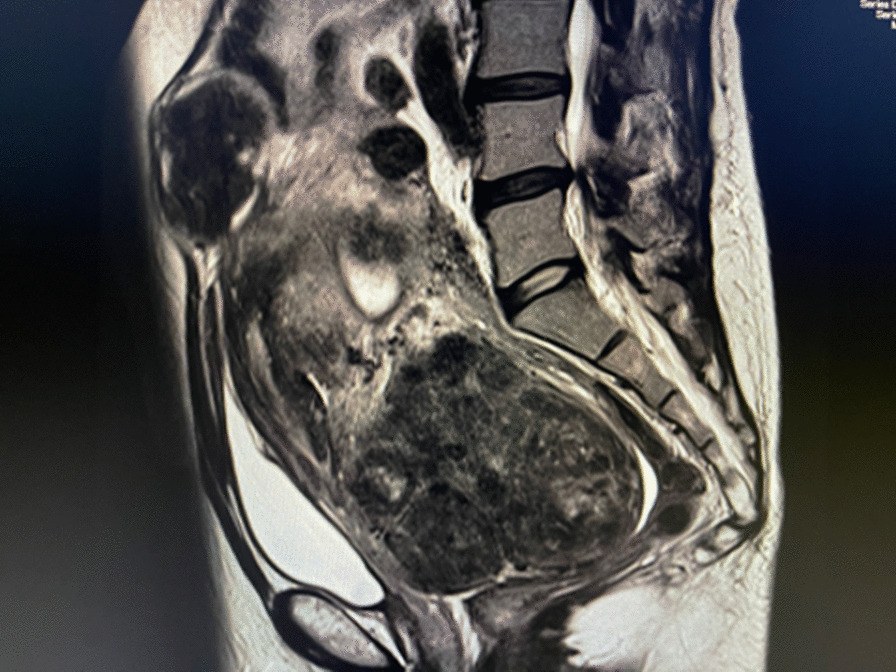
Magnetic resonance images showing an enlarged bicornuate bicollis uterus with diffuse adenomyosis. MRI showing bicornuate bicollis uterus with multiple leiomyomas, diffused adenomyosis, and multiple hematomas in the uterus. Size of the uterus, including fibroid and adenomyosis, measured 22 cm in length and nearly 10 cm in thickness
